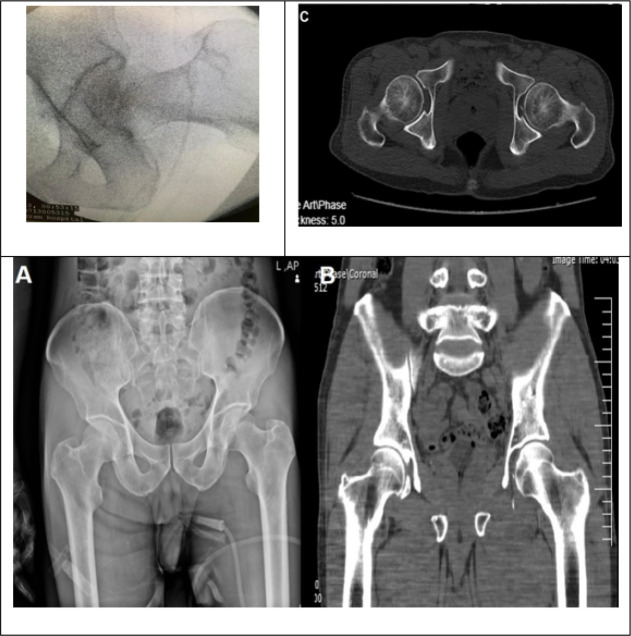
Reduction confirmed by fluoroscopy in the operation room, pelvic X-ray radiograph (A), and coronal (B) and axial (C) views of pelvic computed tomography scan
